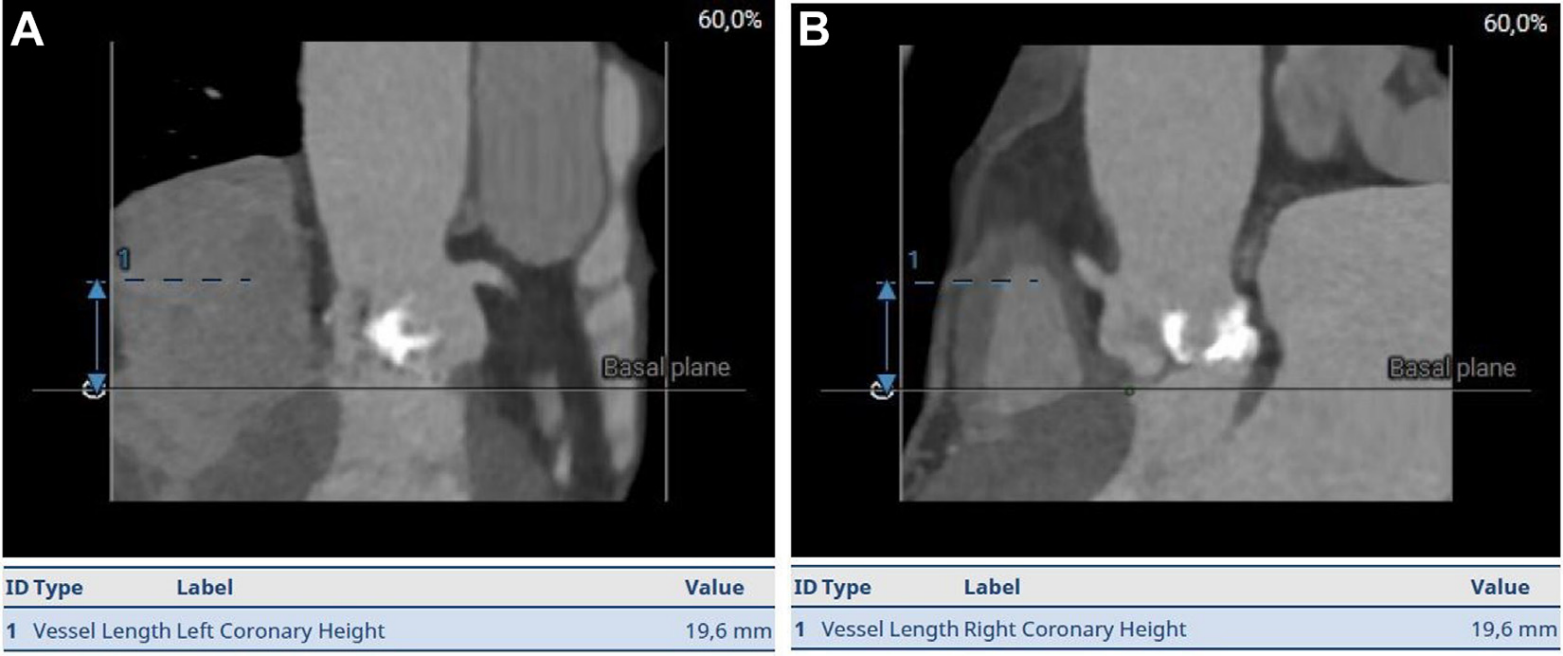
Height of the Coronary Ostia Relative to the Aortic Annular Plane Computed tomography measurement of distance from the aortic annulus to the left (A) and right (B) coronary artery.
